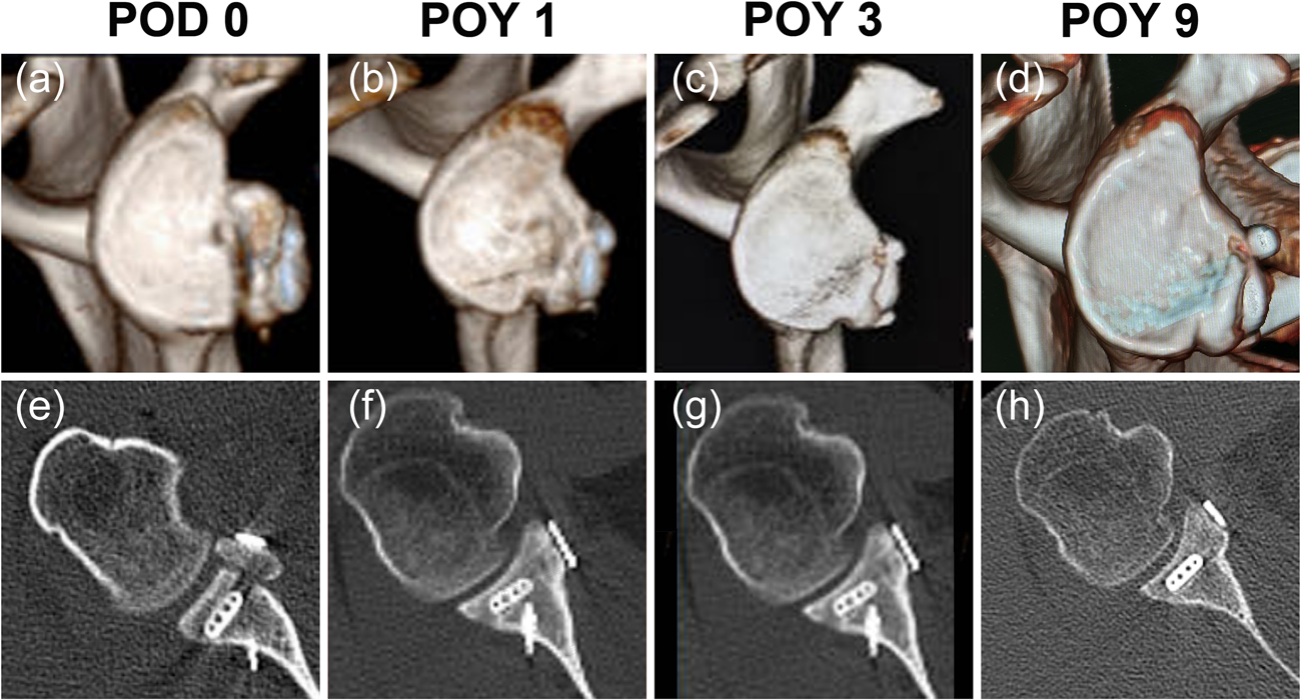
One case (No. 2) of Endobutton misplacement and remodelling of the CG‐glenoid interface of the 3D CT en face view (a–d) and the 2D CT axial view (e–h). 2D, two‐dimensional; 3D, three‐dimensional; CG, coracoid graft; CT, computed tomography; POD, postoperative day; POY, postoperative year.
